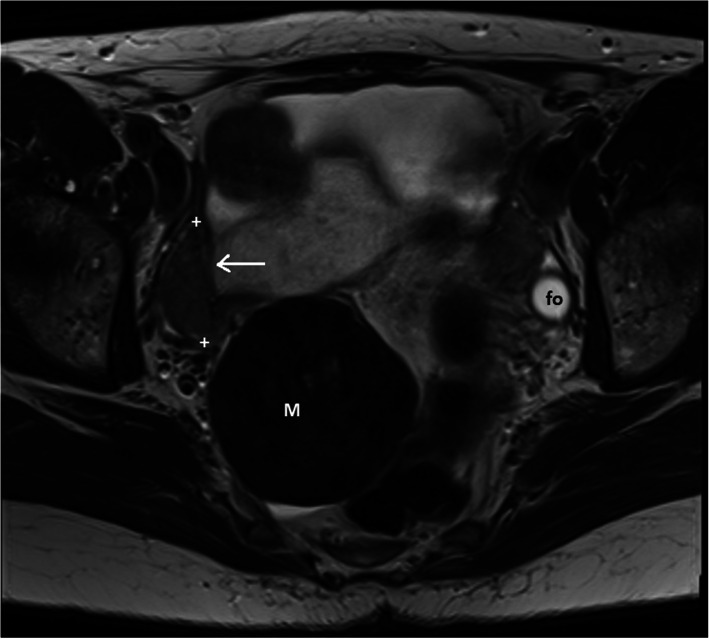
T2 weighted high-resolution MRI in axial plane showing the typical radiological features of a leiomyoma (M) and the uterine remnant at the right side of the patient (arrow). Follicle in the left ovary (fo). MRI was conducted with a 3 T MRI scanner with integrated body coil, spine and body matrix receiving surface coils. Siemens (Skyra Fit, Siemens Medical Solutions, Erlangen, Germany)
